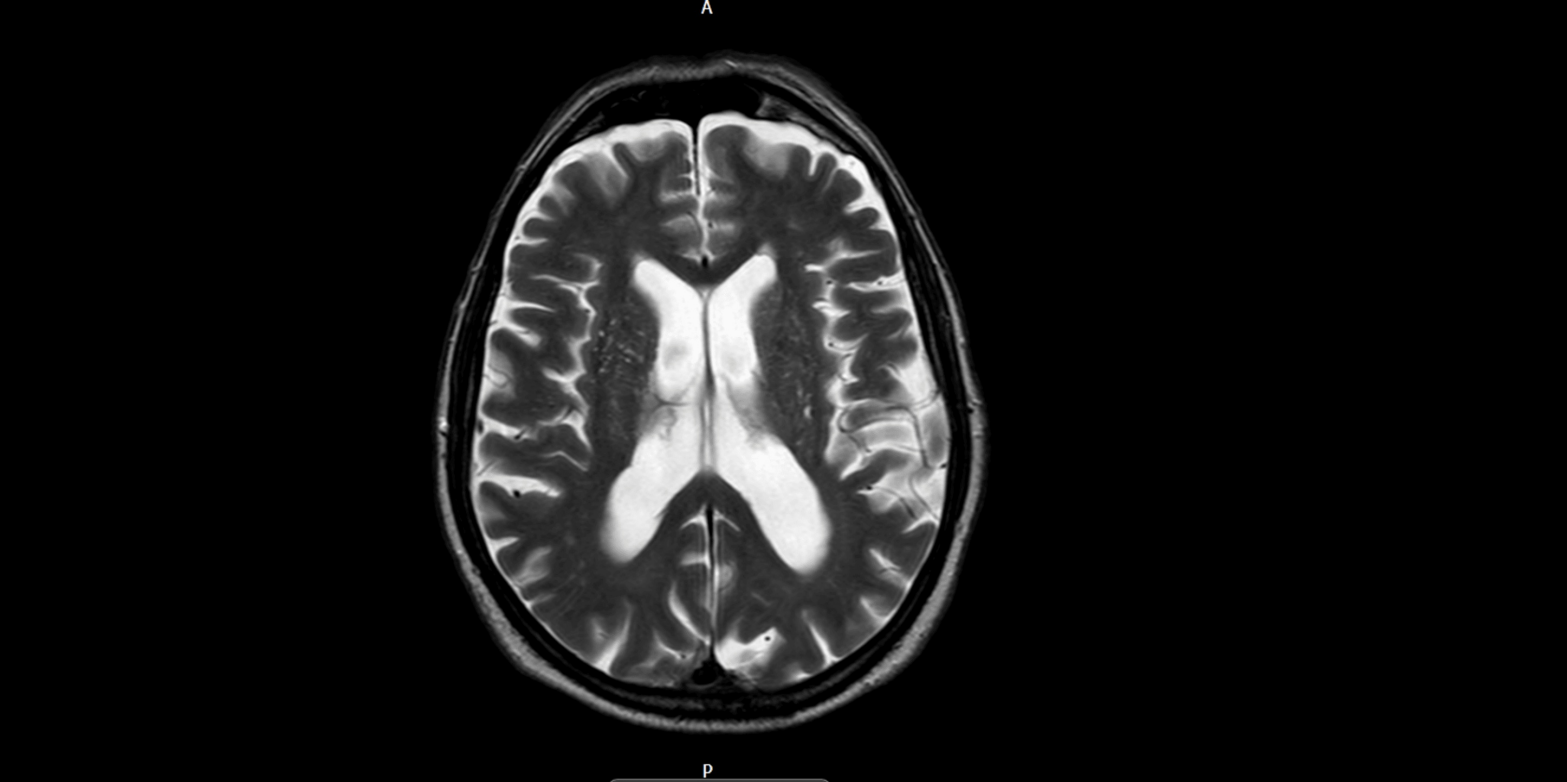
MRI head with contrast (axial T2 view). MRI head (axial T2 view): No acute infarct, hemorrhage, or mass lesion. Normal intracranial vascular flow voids. A small mature cortical infarct is seen in the left middle frontal gyrus on a background of mild chronic small vessel ischemic changes. Foci of microhemorrhage in the right frontal white matter and left occipital lobe are unchanged from previous imaging. The patient has previously been labeled as having multiple transient ischemic attacks during earlier admissions.
